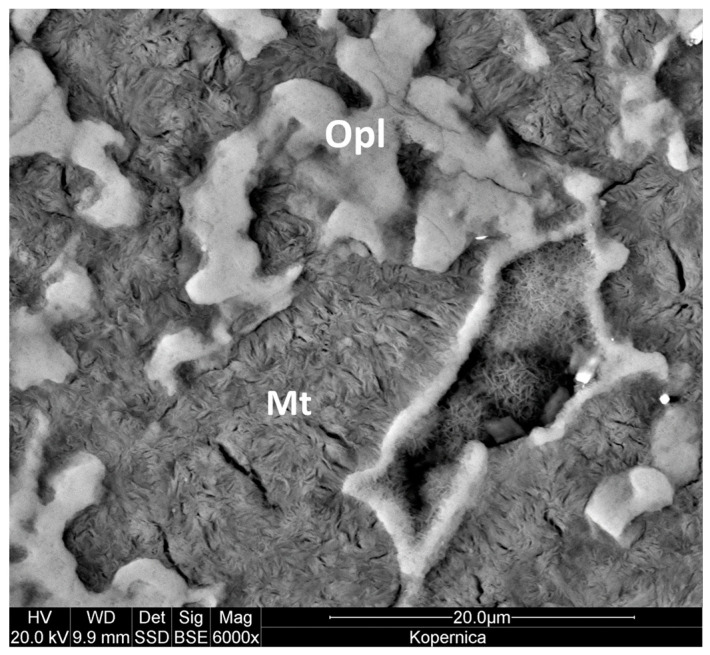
FESEM/BSE image of the polished Kopernica bentonite sample showing montmorillonite (Mt) lumps embedded in opal C/CT (Opl) (areas of greater brightness). For details on mineral identification, see [35].
